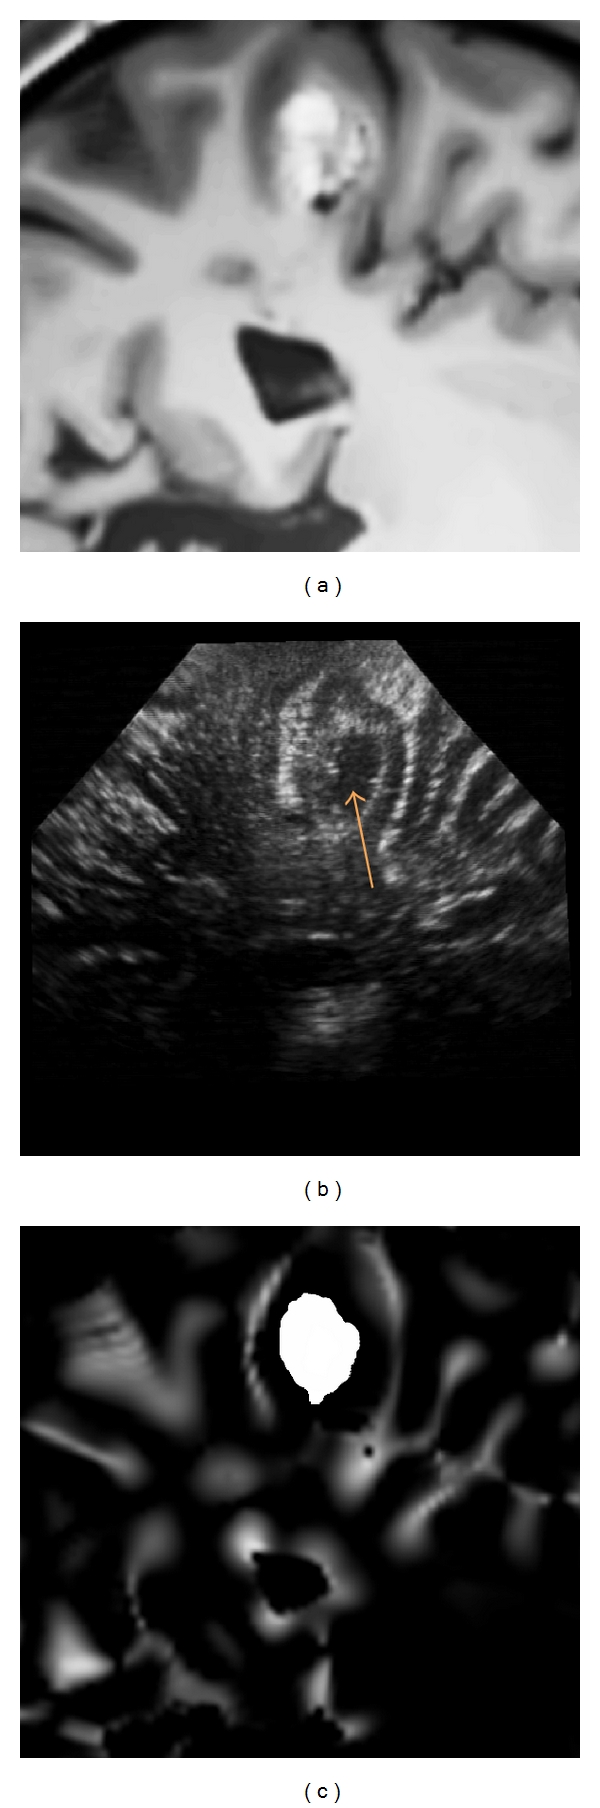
Patient 1. (a) The denoised MR image corresponding to US image (b). (c) The probability map based on *MLvv* operator and extracted from denoising MR image. The preoperative MR image is resliced with the registration matrix provided by the neuronavigation system. The matching is not perfect due to the initial error, estimated around 4.2 mm by the experts for this patient. As visible on the US image, the lesion presents a hypoechogenic central area (indicated on the US image by a narrow).
